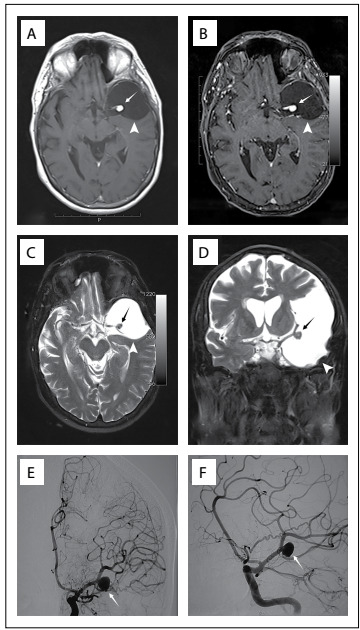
A) Axial T1-weighted non-contrasted magnetic resonance imaging (MRI) showing hypointense lesion at the middle fossa and nodule formation inside the cyst; B) Axial T1-weighted MRI showing hypointense lesion at the middle fossa with saccular dilation inside; C) Axial T2 MRI showing the cyst and the middle cerebral artery bifurcation with saccular dilation at this location; D) Coronal T2 MRI showing the temporal cyst and middle cerebral artery bifurcation with dilation; E) Cerebral arteriography showing aneurysm at the left middle cerebral artery bifurcation; F) Oblique-incidence cerebral angiography showing the aneurysm.
